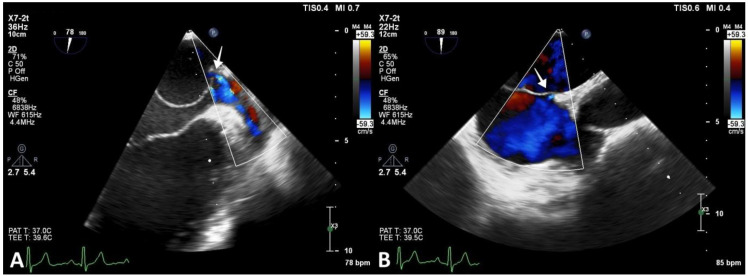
Transesophageal echocardiography revealing CTS. (A) Image showing openings in the CTS membrane (arrow). (B) PFO with minimal spontaneous left-to-right shunt (arrow).
